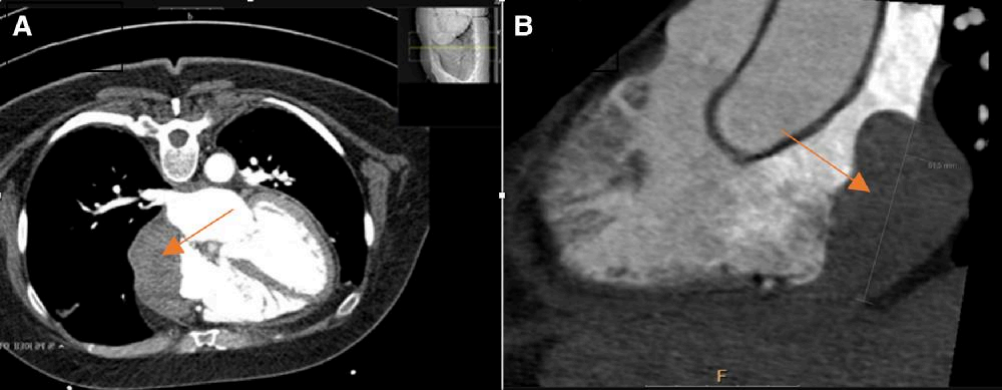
CT scan in axial layering (arterial phase) showing a smooth-bordered, inhomogeneously enhancing lesion of the wall of the right atrium (diffuse intramural growth). (*A*) CT scan in parasagittal view demonstrating the lesion, measuring up to a maximum of 6.2 cm, bulging convexly into the pulmonary trunk (*B*).
